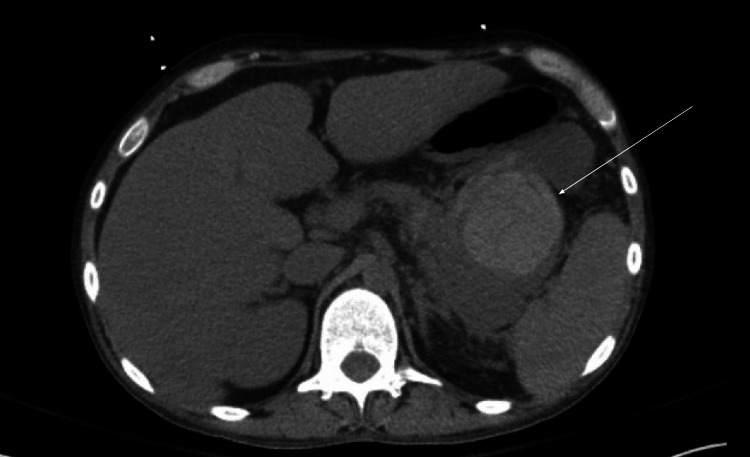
CT abdomen and pelvis on hospital day 1 CT demonstrating a 7.5 cm hyperdense mass-like lesion in the lesser sac, consistent with hemorrhage abutting the stomach, pancreatic tail, and spleen. No evidence of acute pancreatitis or necrotizing process was observed.
